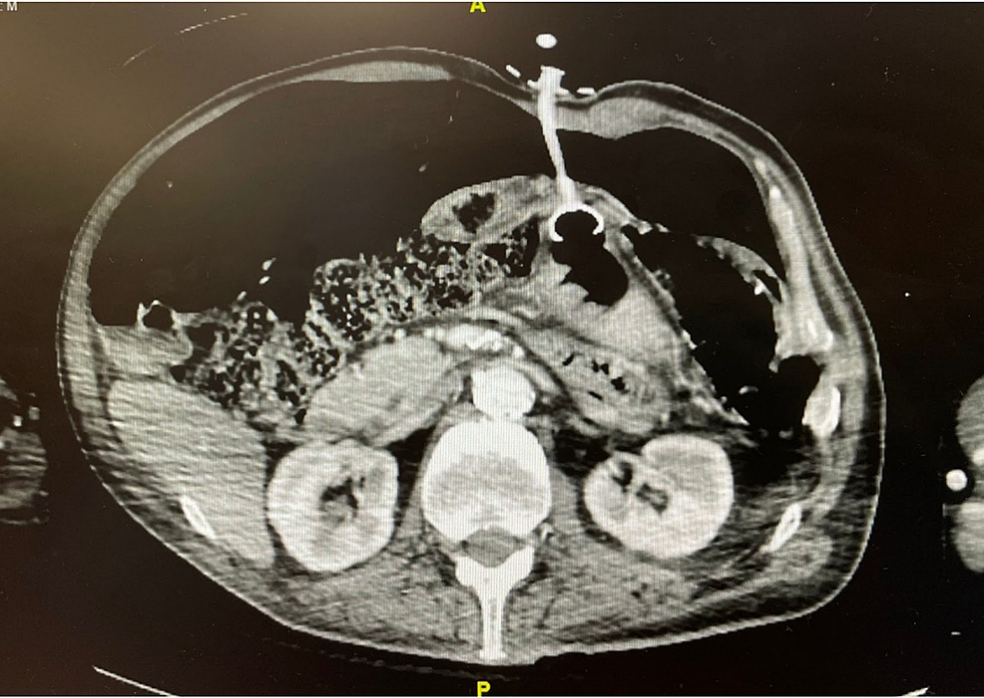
Admission CT abdomen and pelvis demonstrating misplaced PEG tube and pneumoperitoneum. PEG, percutaneous endoscopic gastrostomy
